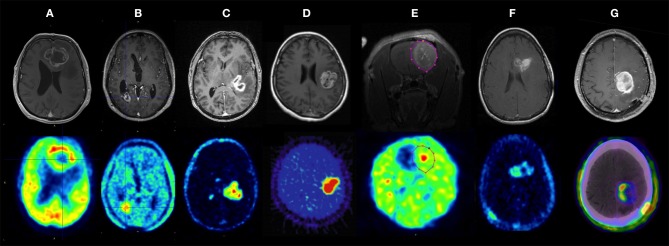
Contrast-enhanced MRI (top row) and multiple PET tracers (bottom row) in glioblastoma. (A)
